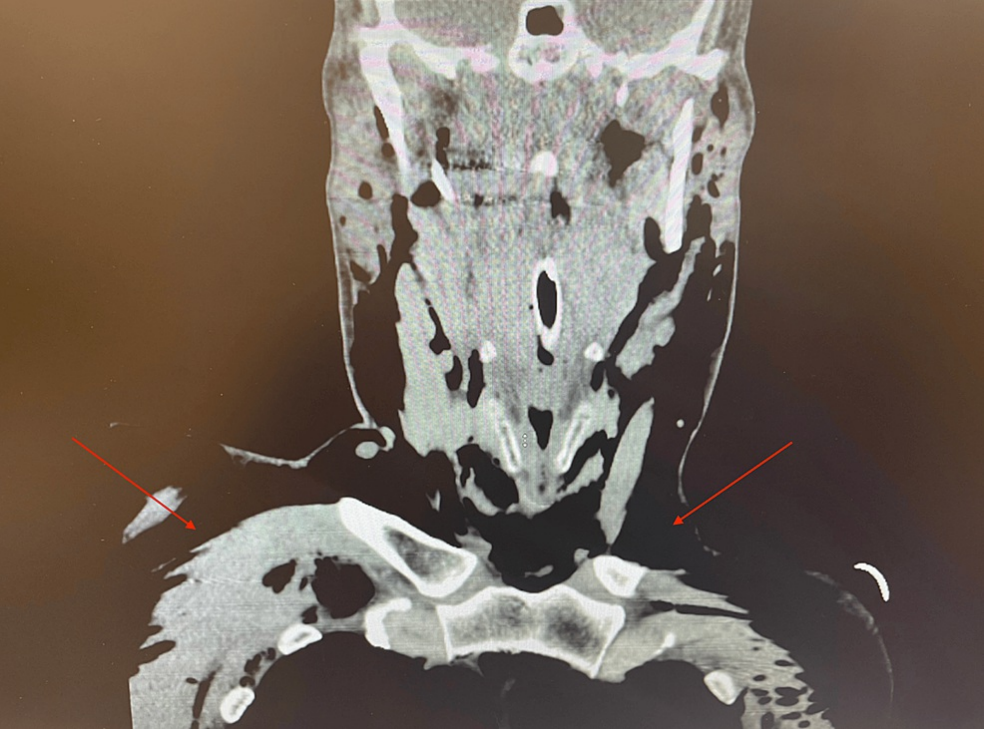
CT scan of the neck obtained postoperatively, which demonstrated extensive soft tissue air without defects in the trachea or esophagus.
